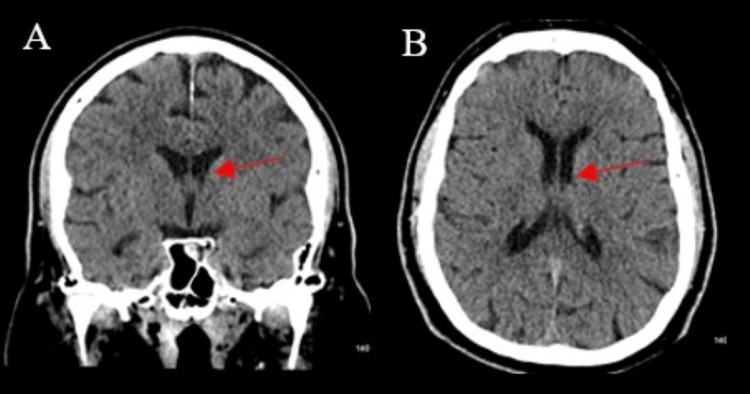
Brain CT at two-month follow-up A: Two-month non-contrast head CT coronal slice demonstrating no hyperdense lesion of the fornix (arrow). B: Two-month non-contrast head CT axial slice demonstrating no hyperdense lesion of the fornix (arrow). CT: computed tomography
